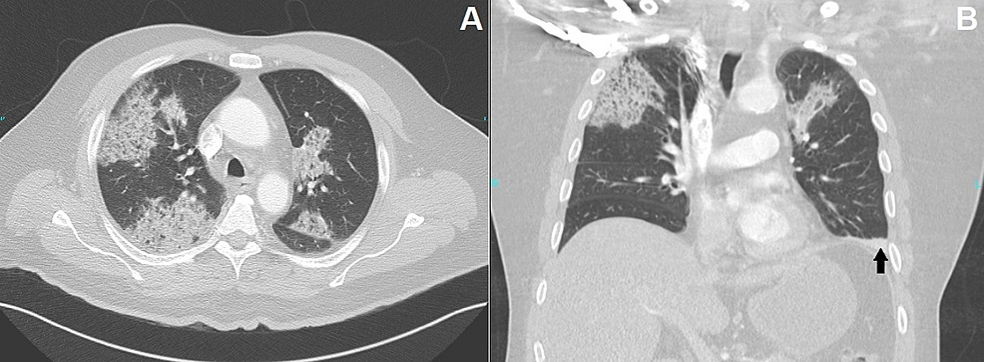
Axial (A) and coronal (B) enhanced chest CT slices in lung window Patchy consolidation areas can be seen in both upper lung lobes. Additionally, there is a subpleural atelectatic change seen in the left lung base (Black arrow in B).
